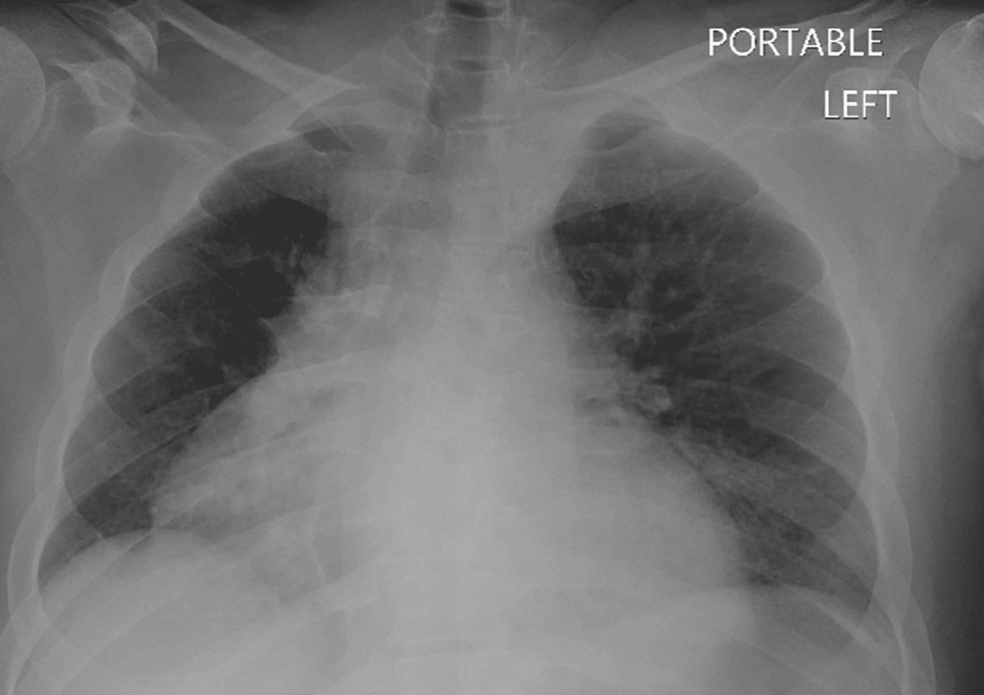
Initial chest X-ray showing the enlarged heart
